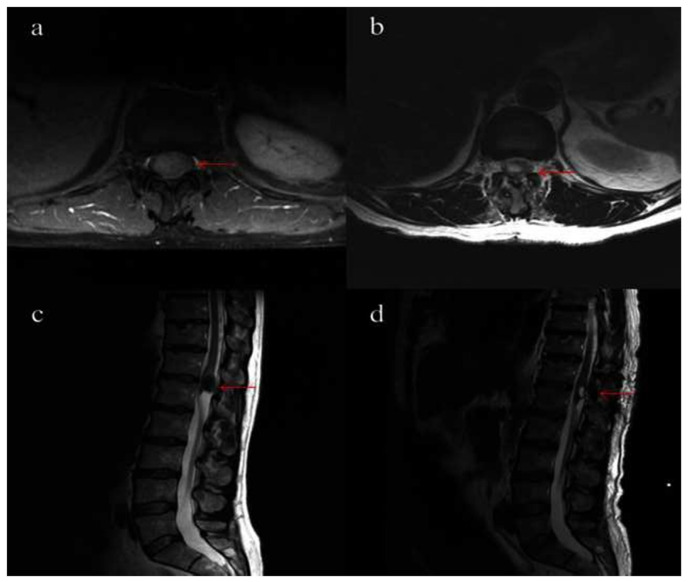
Spinal MRI of case 3. Preoperative axial T1-weighted (a) and sagittal T2-weighted (c) MRI. Postoperative axial (b) and sagittal (d) T2-weighted MRI.
